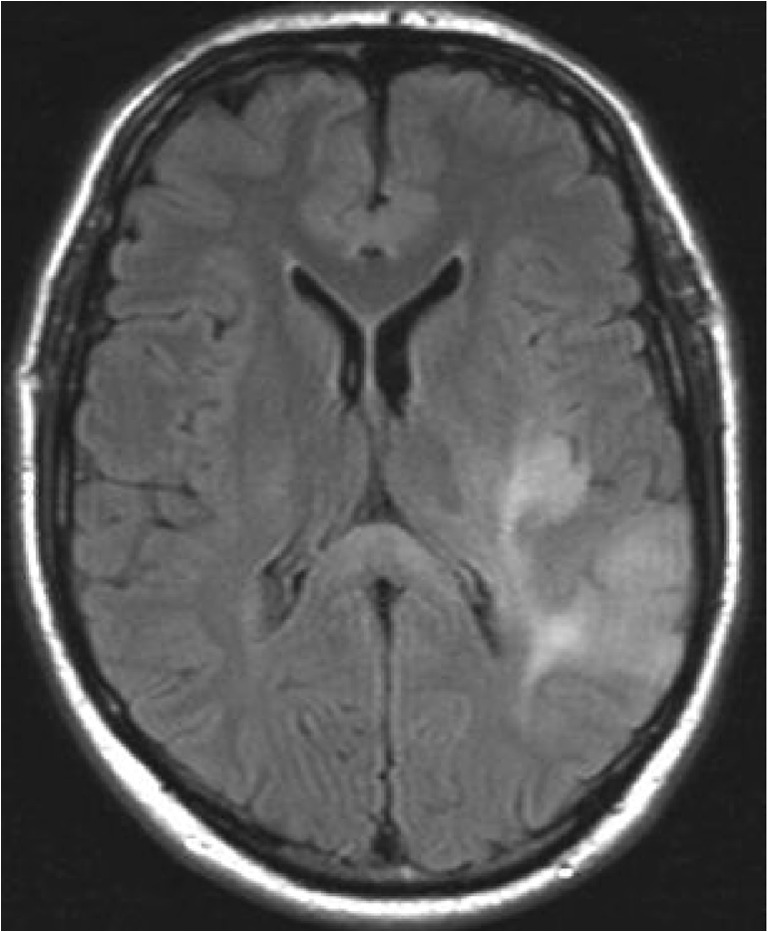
Forty-nine-year-old man with a diffuse glioma grade 2. Axial FLAIR shows a diffuse tumor with extension into the splenium, consistent with appearance of gliomatosis cerebri
